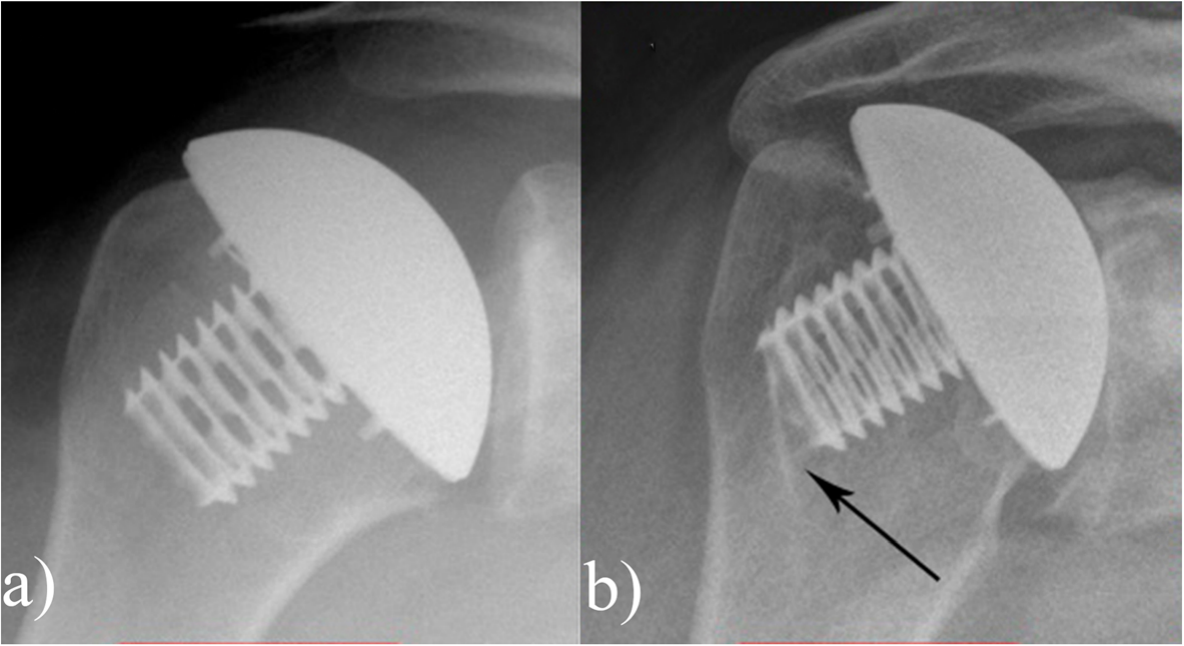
a Post operative image post surgery, b radiograph at six years showing stress shielding around the coring screw indicated by the arrow
